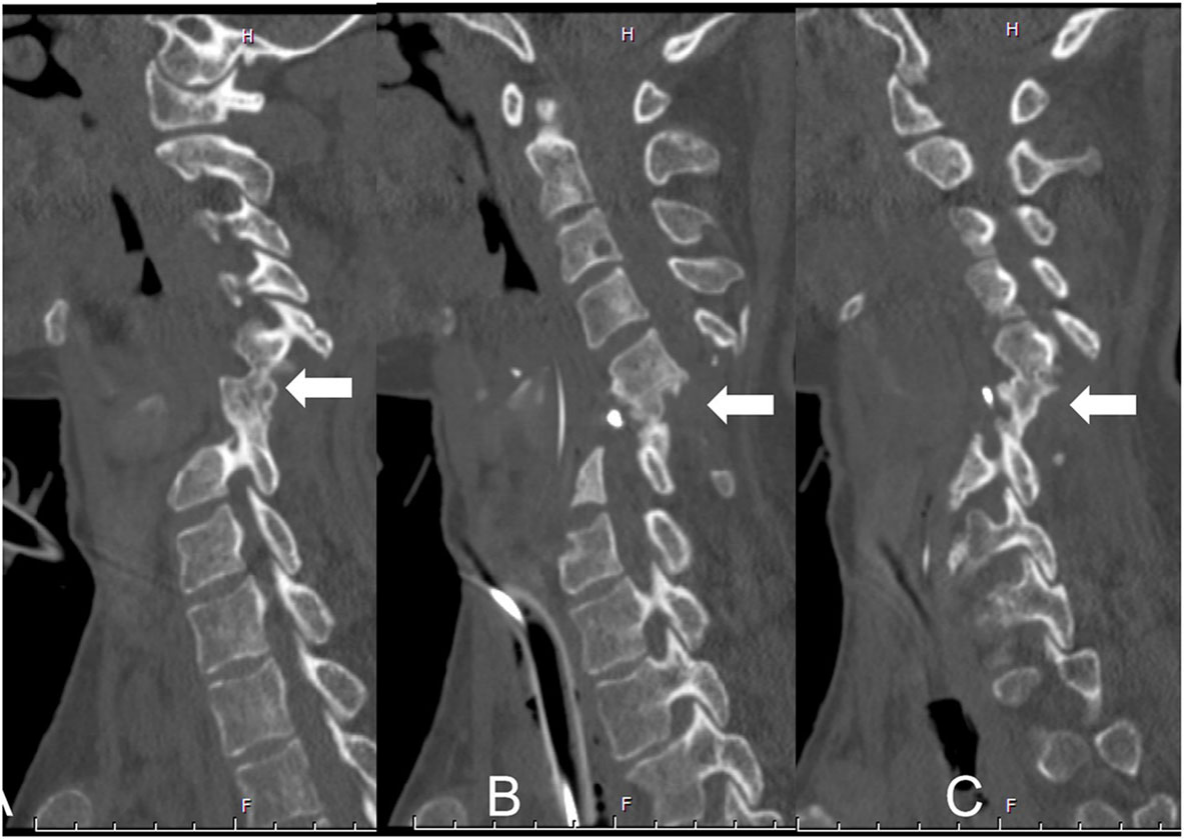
Cervical CT nine months post injury showed fixed malunion of the cervical spine (demonstrated by the arrows)
